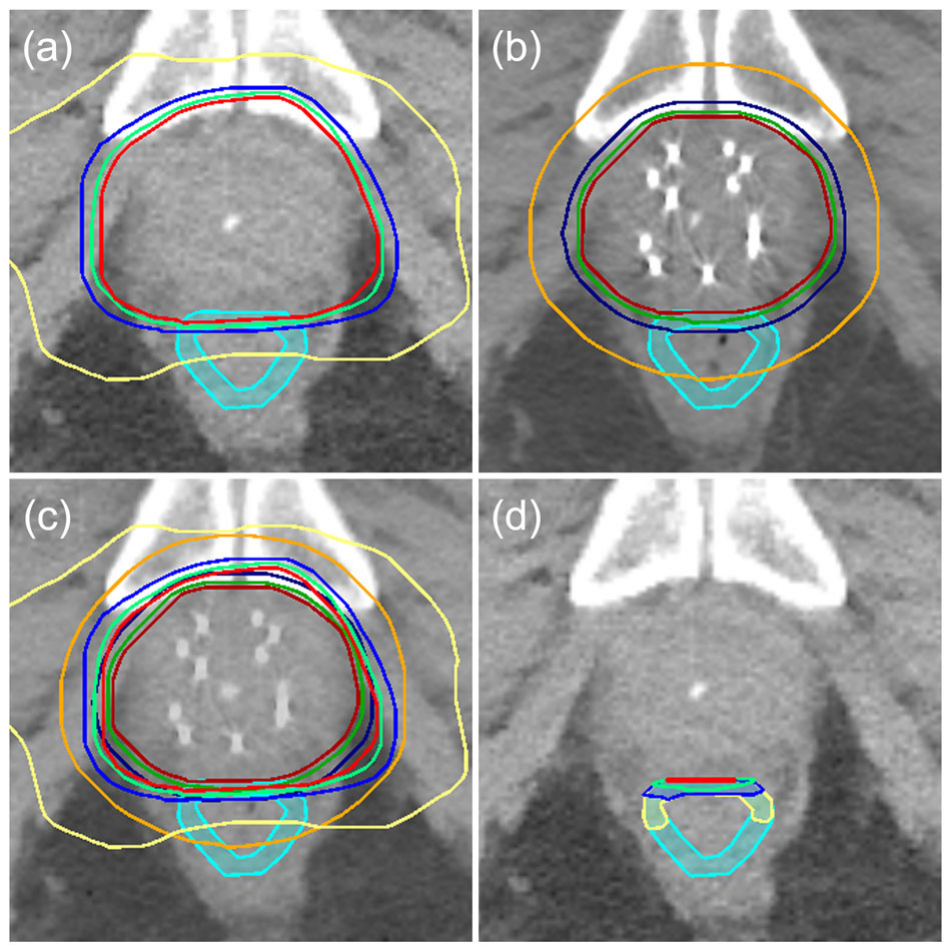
An example of computed tpmpgrapy (CT) image depicts isodose lines in volumetric modulated arc therapy (VMAT) and prostate seed implant (PSI). (a) In VMAT plan, volumetric dose *D*
